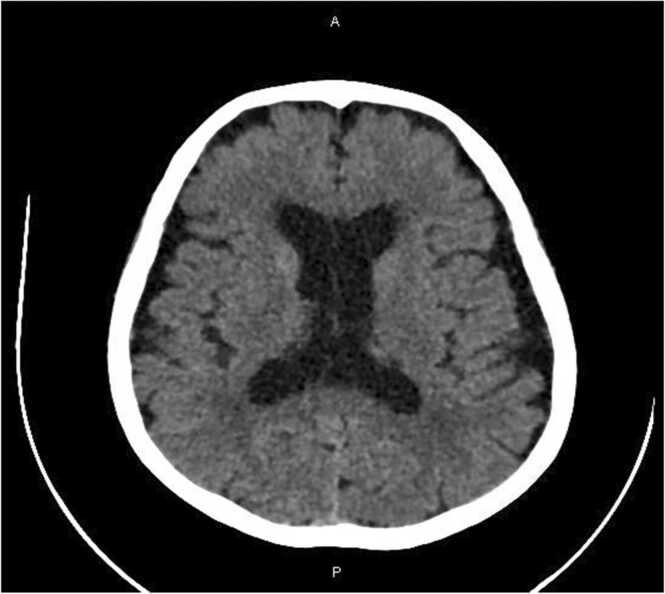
CT head: showed Age-related parenchymal involutional changes including dilated ventricular system.
